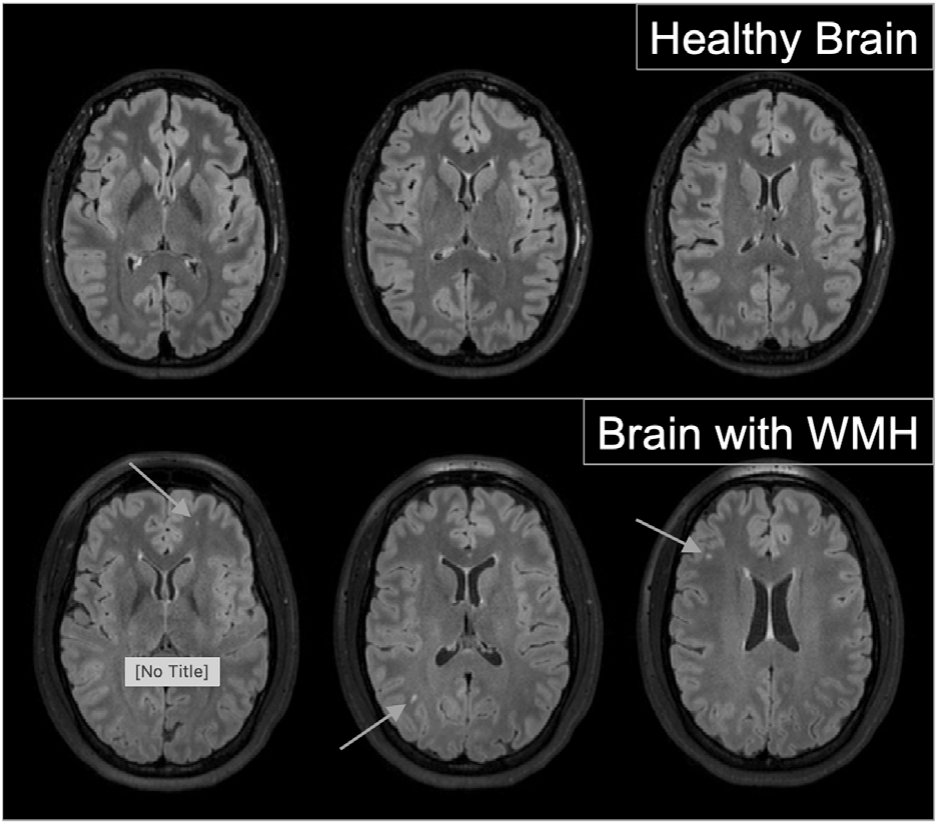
Examples of healthy brain images (top panel) and images from brains with evidence of which matter hyperitensities (bottom panel) from fluid-attenuated Inversion Recovery (FLAIR) magnetic resonance imaging.
